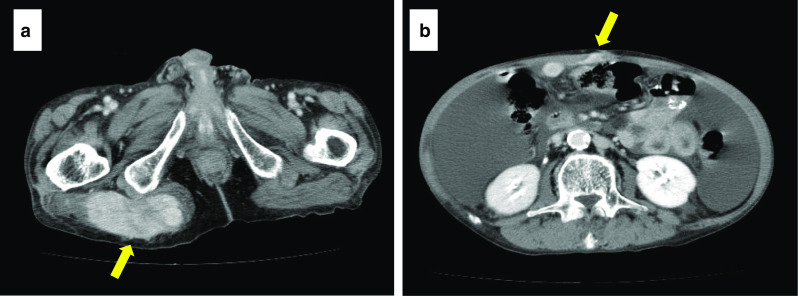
Abdominal contrast-enhanced computed tomography images 8 months after gastrectomy. a Enlarged right gluteal muscle metastasis (arrows). b Disseminated nodule on the abdominal wall (arrows) and increased ascites can be observed
